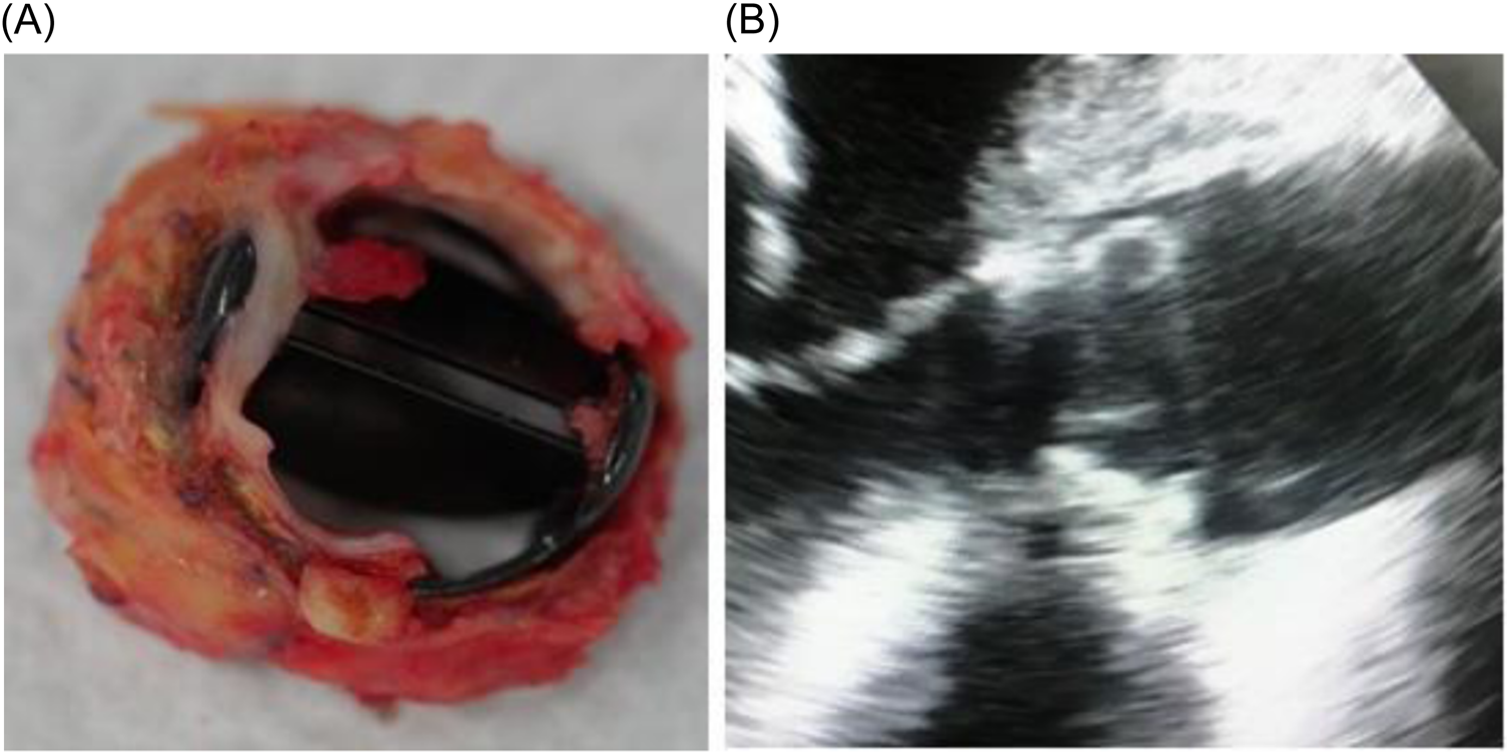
Previous 19 mm St. Jude mechanical aortic valve. (A) Surgical view with pannus and thrombus formation. (B) Echocardiographic view
